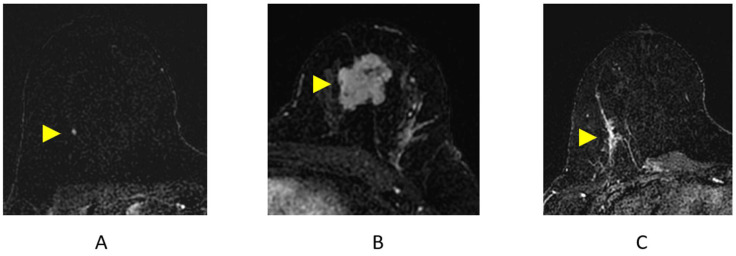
Breast MRI Findings on axial post-contrast subtraction breast MRI (arrowheads). (A) Focus; (B) mass; (C) non-mass enhancement (NME).
